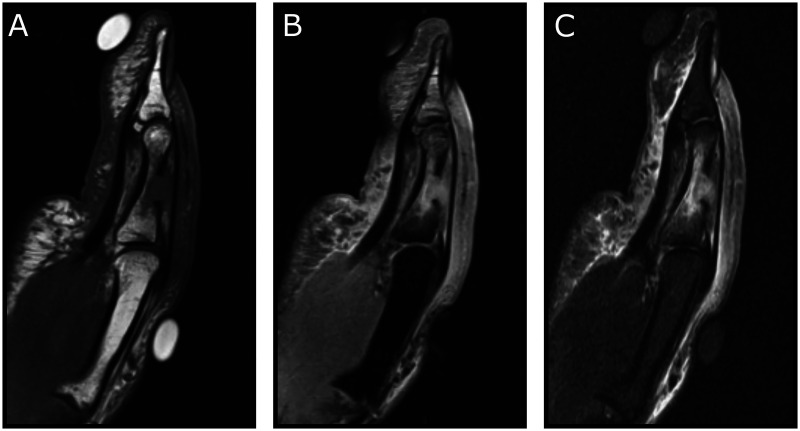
Lateral T1-weighted (A), T2-weighted (B), and STIR (C) sequences of the left thumb MRI showing cortical permeation, in continuity with a subcutaneous panniculitis and phlegmonous changes.
